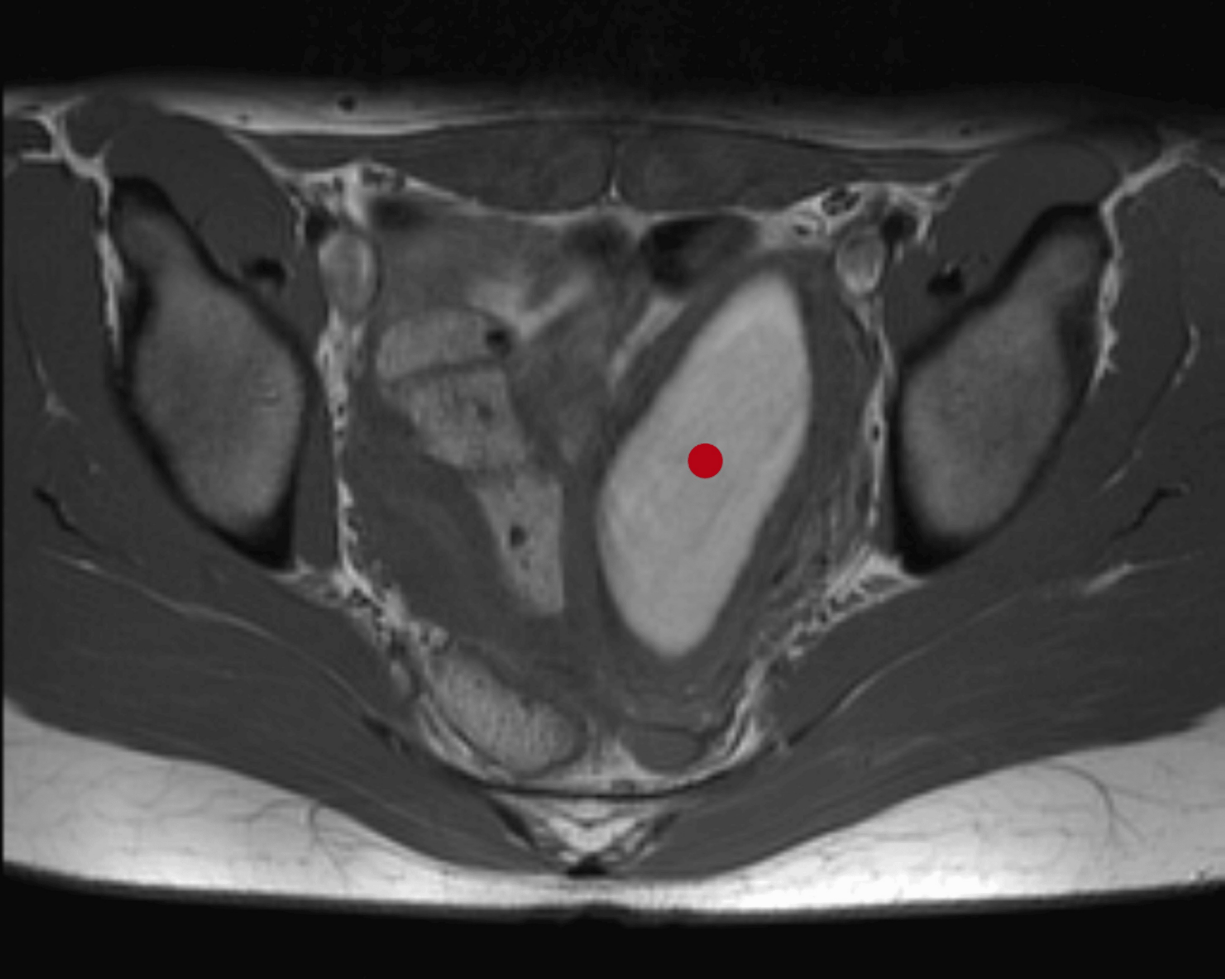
Axial T2 MRI Red dot: distended left uterine cavity MRI: magnetic resonance imaging
